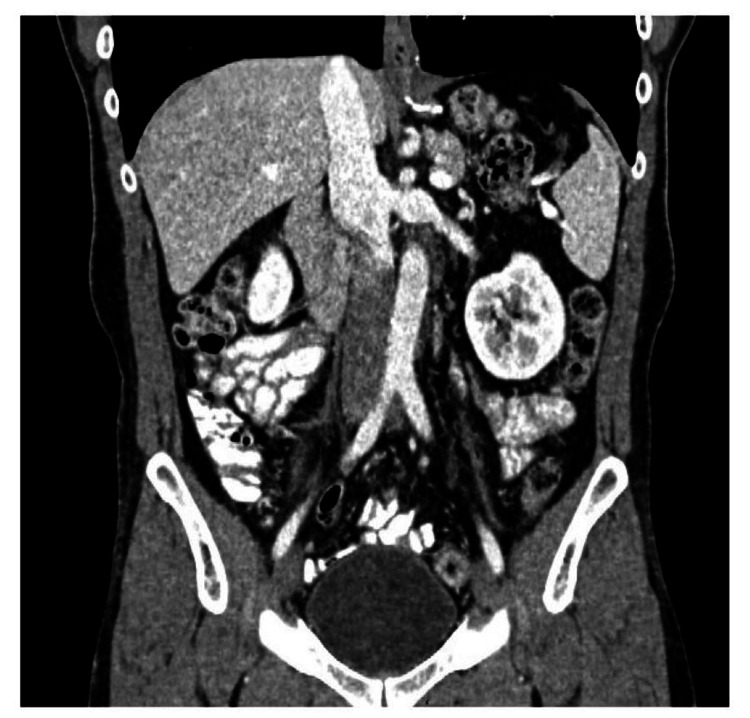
The CT scan of the abdomen showed resolution of the lymph nodes following adjuvant chemotherapy.
